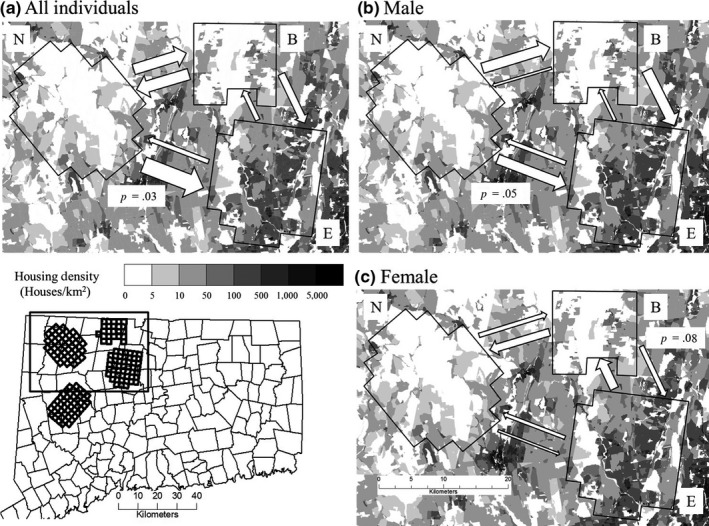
Black bear migration rates among all individuals, males, and females between study areas in northwest CT. Arrow sizes are proportional to first‐generation migration rates estimated by GENECLASS 2. Significant (*p* < .05) probabilities of asymmetry estimated by nonparametric chi‐square contingency tests are displayed for pairwise comparisons of migration rates between North (N), East (E), and Barkhamsted (B) grids
